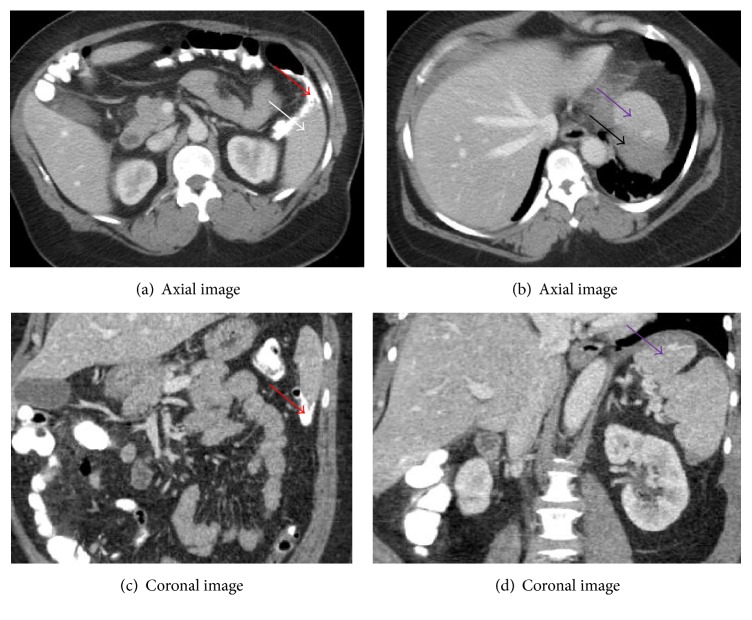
CT, 05/05/17: perisplenic and subcapsular hematoma (black arrow) along the dome of the spleen, with an area of active bleeding along the superior subcapsular region (purple arrows) with no clear fat plane separation between the colonic splenic flexure and the lower portion of the spleen (white arrows) and contrast tracking irregularly along diverticula/splenocolic adhesions and splenocolic ligament (red arrows).
